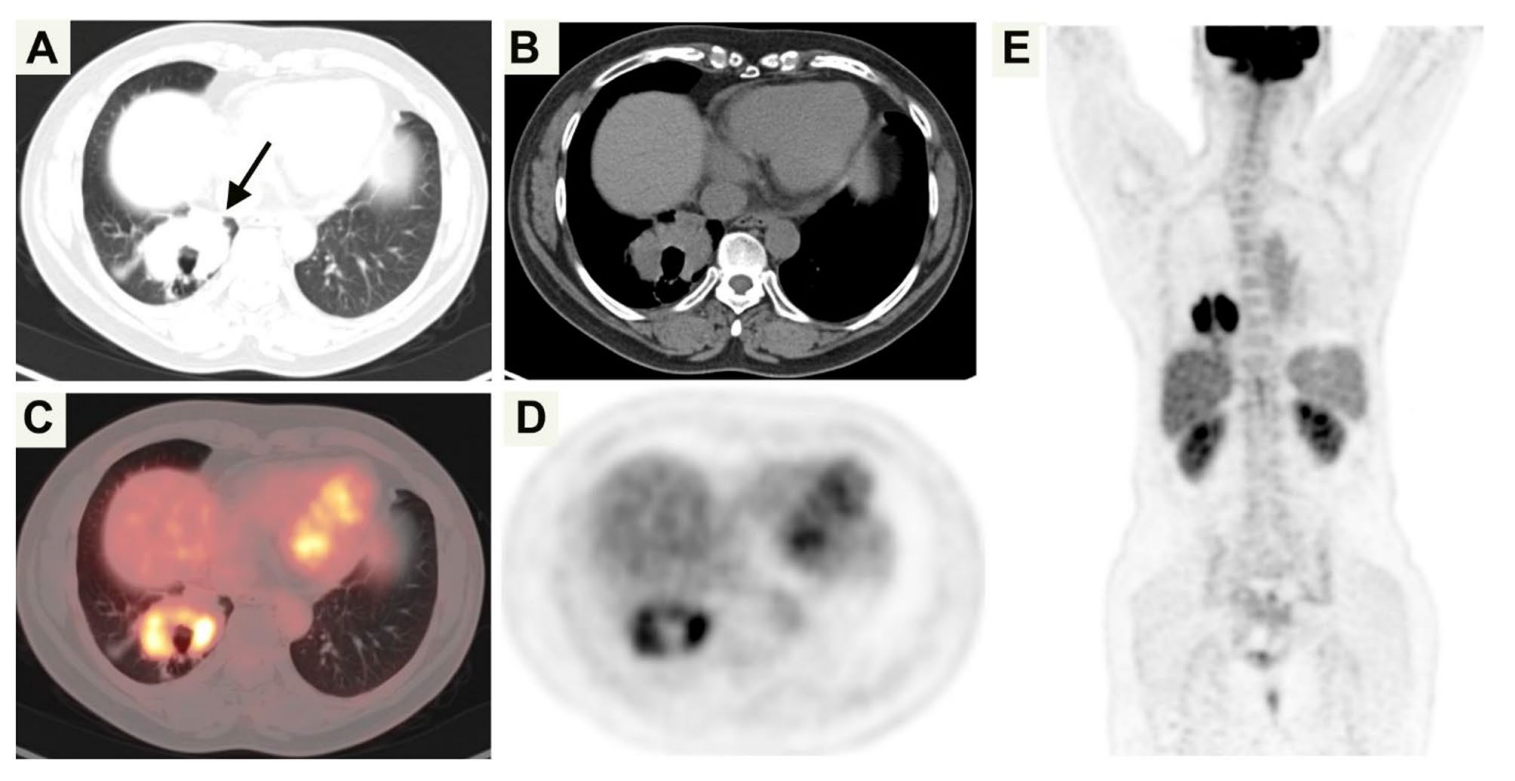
^18^ F-FDG PET/CT images. Right lung lesion showed an irregular mass, central cavity and annular marked FDG uptake with maximum standardized uptake value (SUVmax) of 20.7 (black arrow); (A) Axial lung window; (B) Axial soft tissue window ; (C) PET/CT fusion image;(D) PET ; (E) Maximum intensity projection image of whole body
